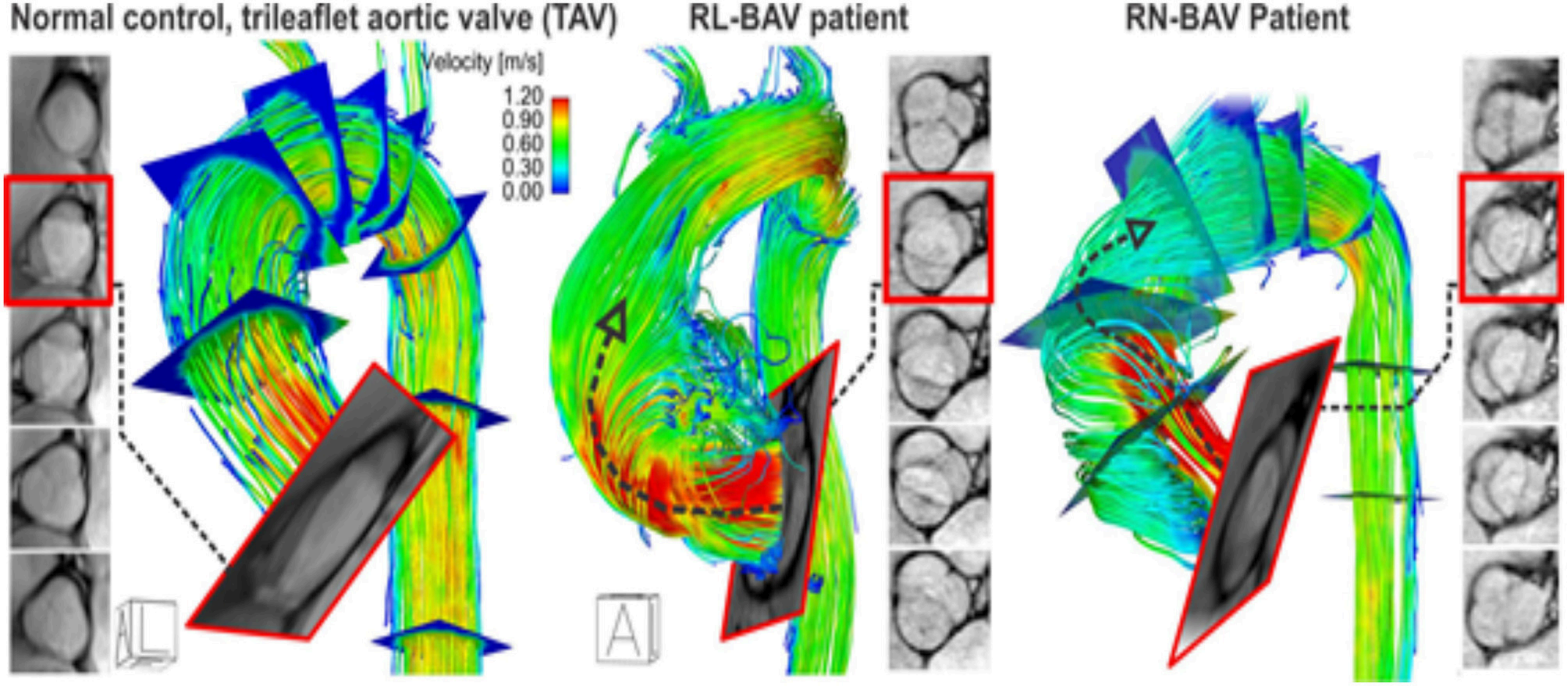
4D flow MRI in a control and BAV patient with a right-left (RL) fusion pattern and right-non-coronary (RN) fusion pattern. Note that the RL-BAV exerted significant eccentric aortic outflow jet (but not higher velocity, arrow) compared to TAV. The BAV phenotype (RL vs. RN) greatly influences aortic outflow, which in turn, affects aortic segments exposed to elevated WSS (Figure 4). Adapted with permission (Barker et al., 2012; Guzzardi et al., 2015).
